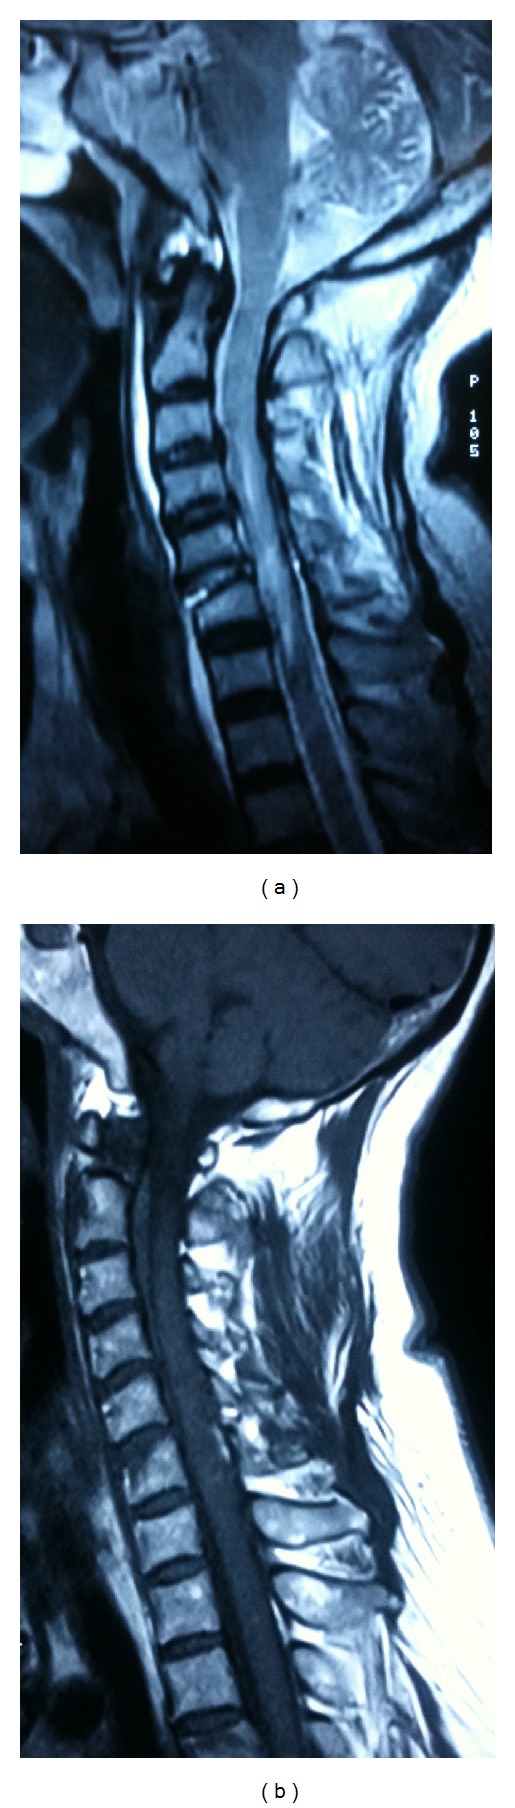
MRI of the patient. (a) T2-weighted image showing high signal intensity area at C5/6 intervertebral space. (b) T1-weighted image showing low signal intensity area at C5/6 intervertebral space.
